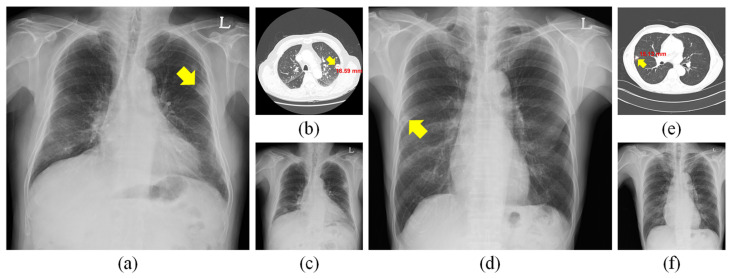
Initial and follow-up CXRs and CT images with a pulmonary lesion. (a) Initial CXR of a 65-year-old male patient with metastatic renal cell carcinoma in the left upper lobe and (b) CT examination of the patient (a). (c) Follow-up CXR of (a). (d) Initial CXR of an 81-year-old male patient with metastatic adenocarcinoma in the right middle lobe and (e) CT examination corresponding to (d). (f) Follow-up CXR of (d). The yellow arrows indicate the localization of suspected pulmonary lesions [16].
